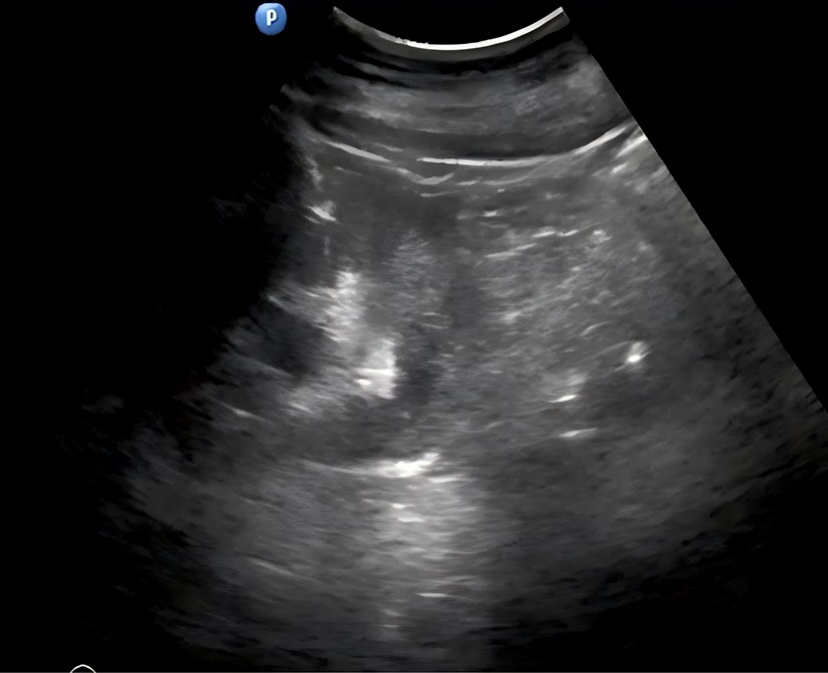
Ultrasound image of percutaneous renal puncture. Ultrasound image of establishing F18 percutaneous access to monitor the puncture route with the assistance of one step balloon dilation
